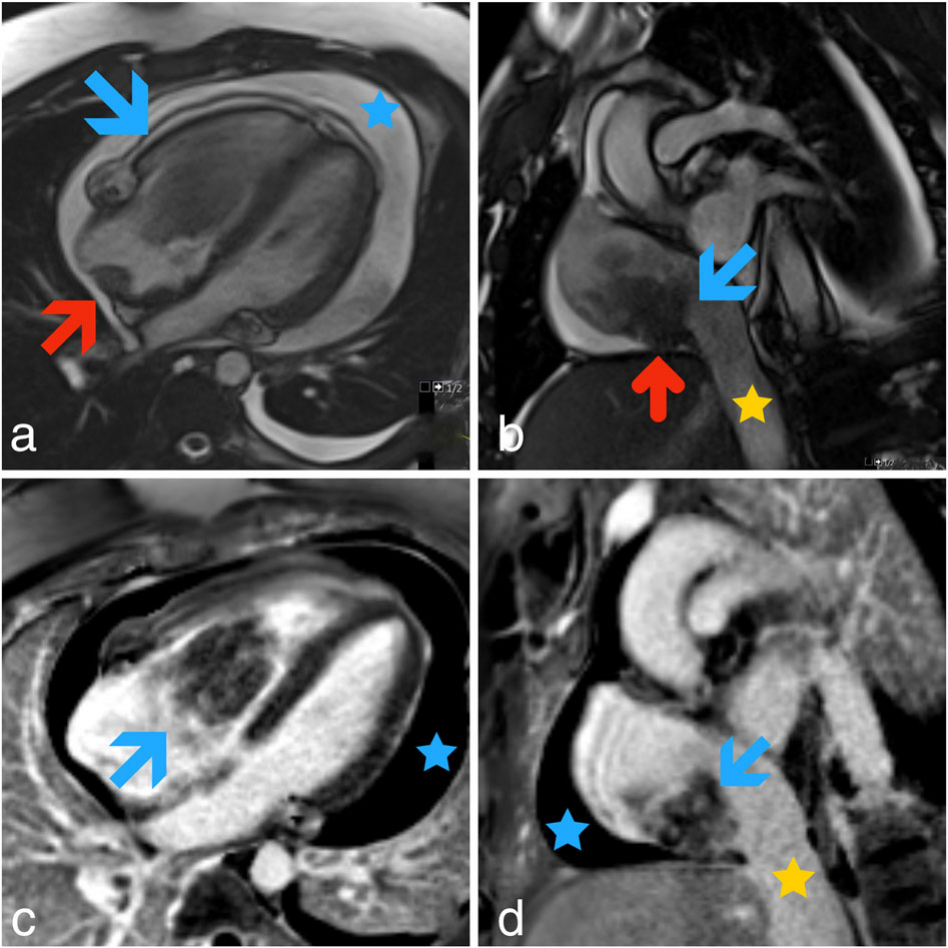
(a) A four‐chamber balanced steady state free precession (bSSFP) cardiac MRI image showing a large frond‐like mass centered in the right atrium seen prolapsing into the right ventricle (blue arrow). The posterior right atrial wall attachment is also shown (red arrow). (b) The corresponding short‐axis bSSFP image through the basal right atrium clearly shows the inferior attachment of the mass (blue arrow) as well as minimal extension into the pericardial space (red arrow). (c and d) Four‐ and two‐chamber phase‐sensitive inversion recovery (PSIR) delayed contrast‐enhanced demonstrate heterogeneous enhancement within the mass (blue arrows) typical of a neoplastic lesion. The yellow star indicates the inferior vena cava, and the blue arrow denotes pericardial effusion
